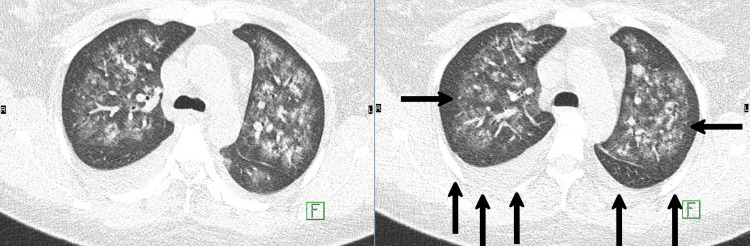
Computed tomography (CT) scan of the chest Axial CT chest at the level of the carina demonstrated extensive ground glass opacities bilaterally with subpleural sparing and small-sized pleural effusion bilaterally right greater than the left.
